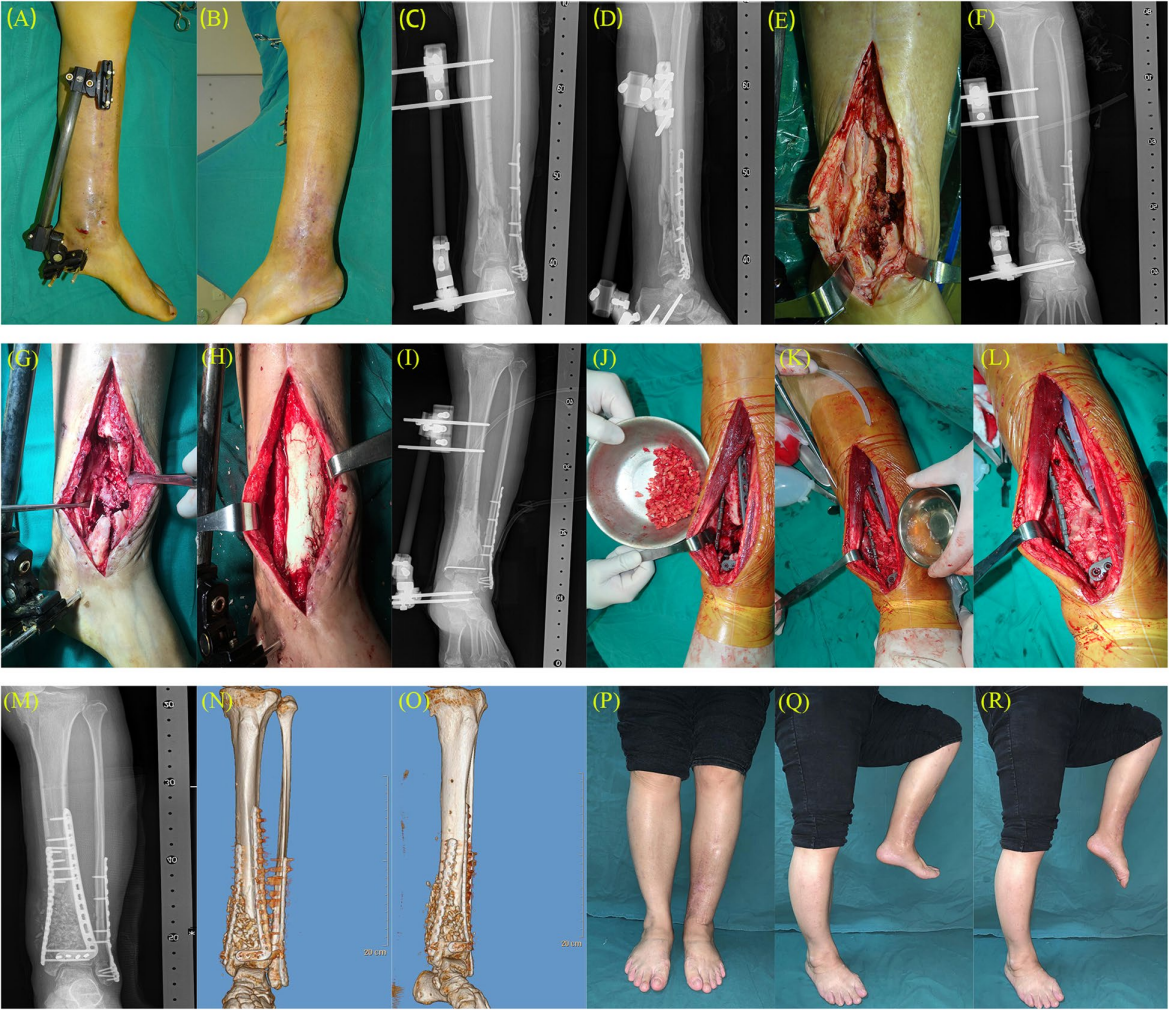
A 44-year-old woman’s left leg was injured by a heavy object, resulting in fracture of the tibia and fibula and fluid exudation for a period of over 3 months. A/B/C/D Appearance and X-ray of the patient's left calf upon admission. E/F Left calf expansion wound and postoperative X-ray. G/H/I One week later, the tibial bone defect was filled using cement spacer. J/K/L Six weeks later, the induced membrane was cut longitudinally and the cement spacer was removed. The distal tibia was fixed with double plates, and iliac bone was removed to make bone granules, which were mixed with PRP and packed into the defective tibial region(K Using PRP). M/N/O X-ray and CT reconstruction show bone reconstruction after bone grafting. P/Q/R 21 months after bone grafting, the foot and ankle function recovered well.The Iowa ankle score was 94
